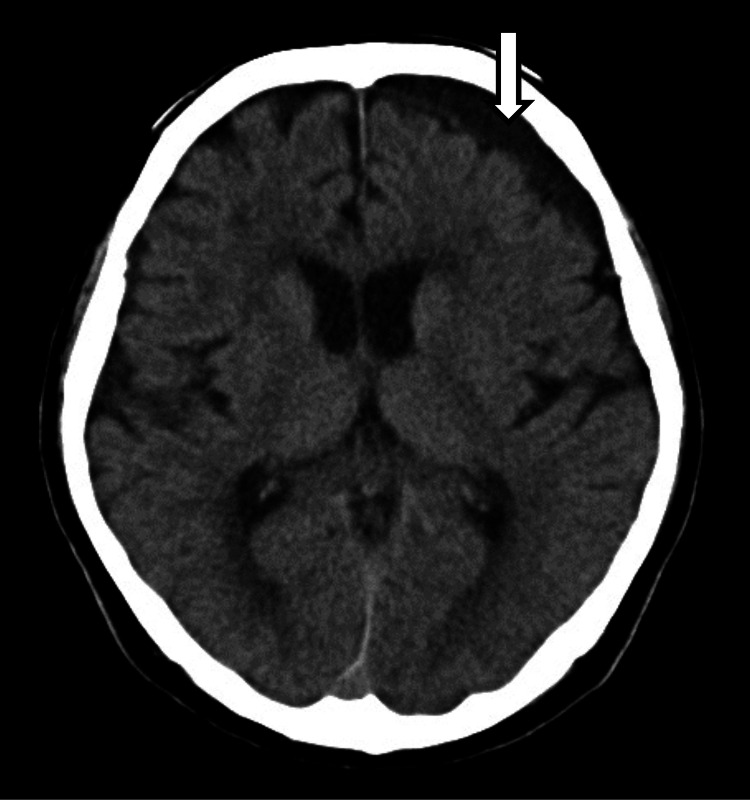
Head CT obtained 13 days after admission showing chronic subdural hematoma on the left (white arrow).
